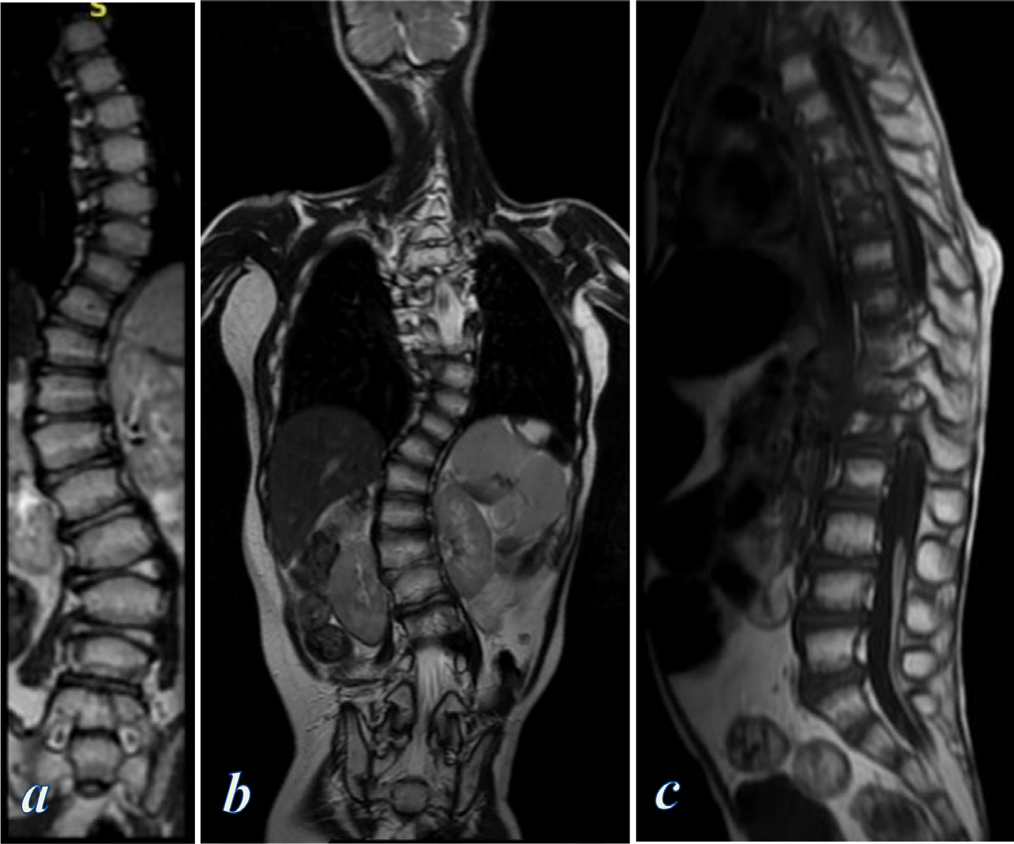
MRI examination of the spine. (a) Coronal *T*
